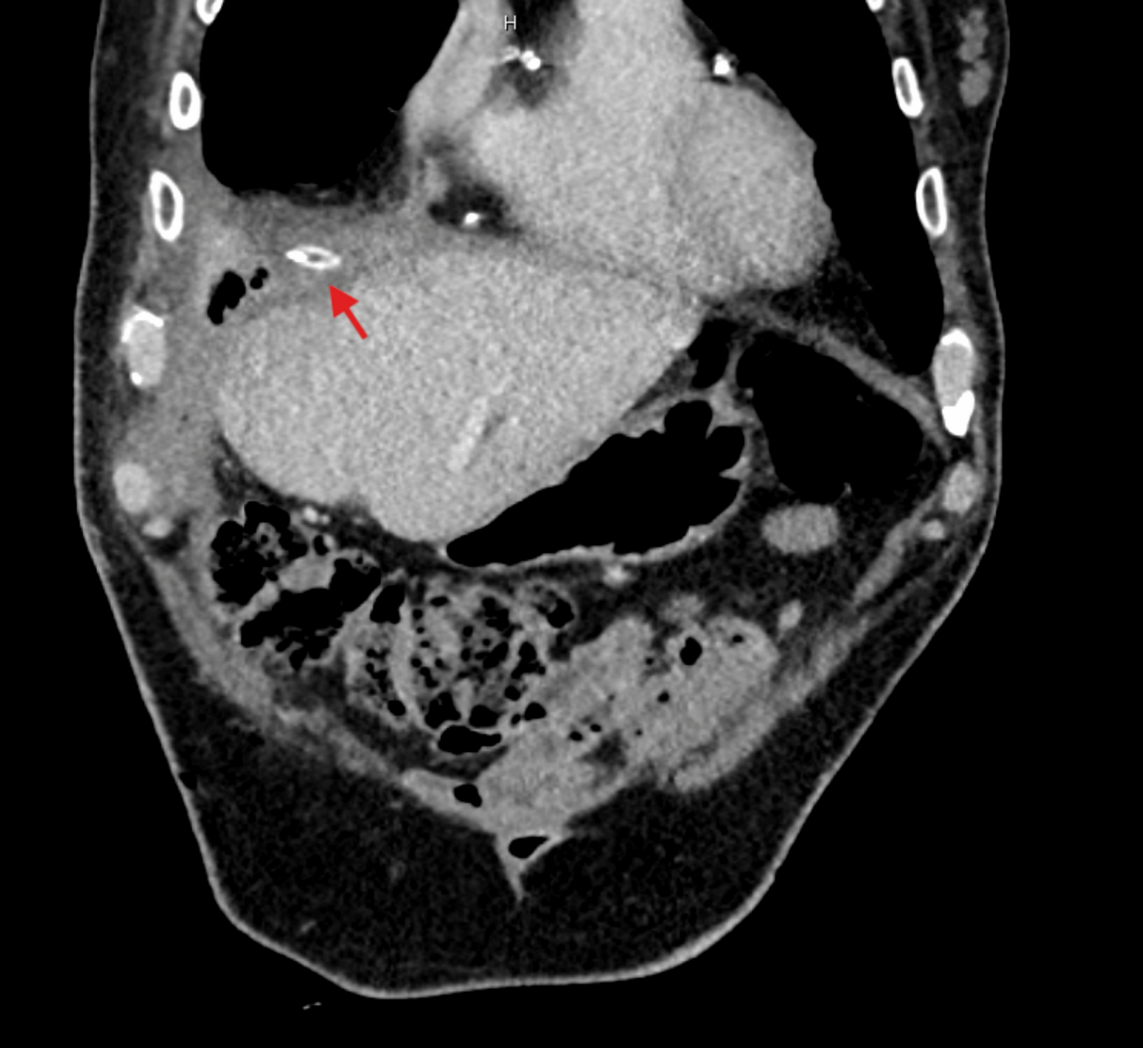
Post-operative CT of the abdomen and pelvis showing a surgical drain (marked with a red arrow), placed in anterior aspect of the liver within the subdiaphragmatic collection. CT: computed tomography.
